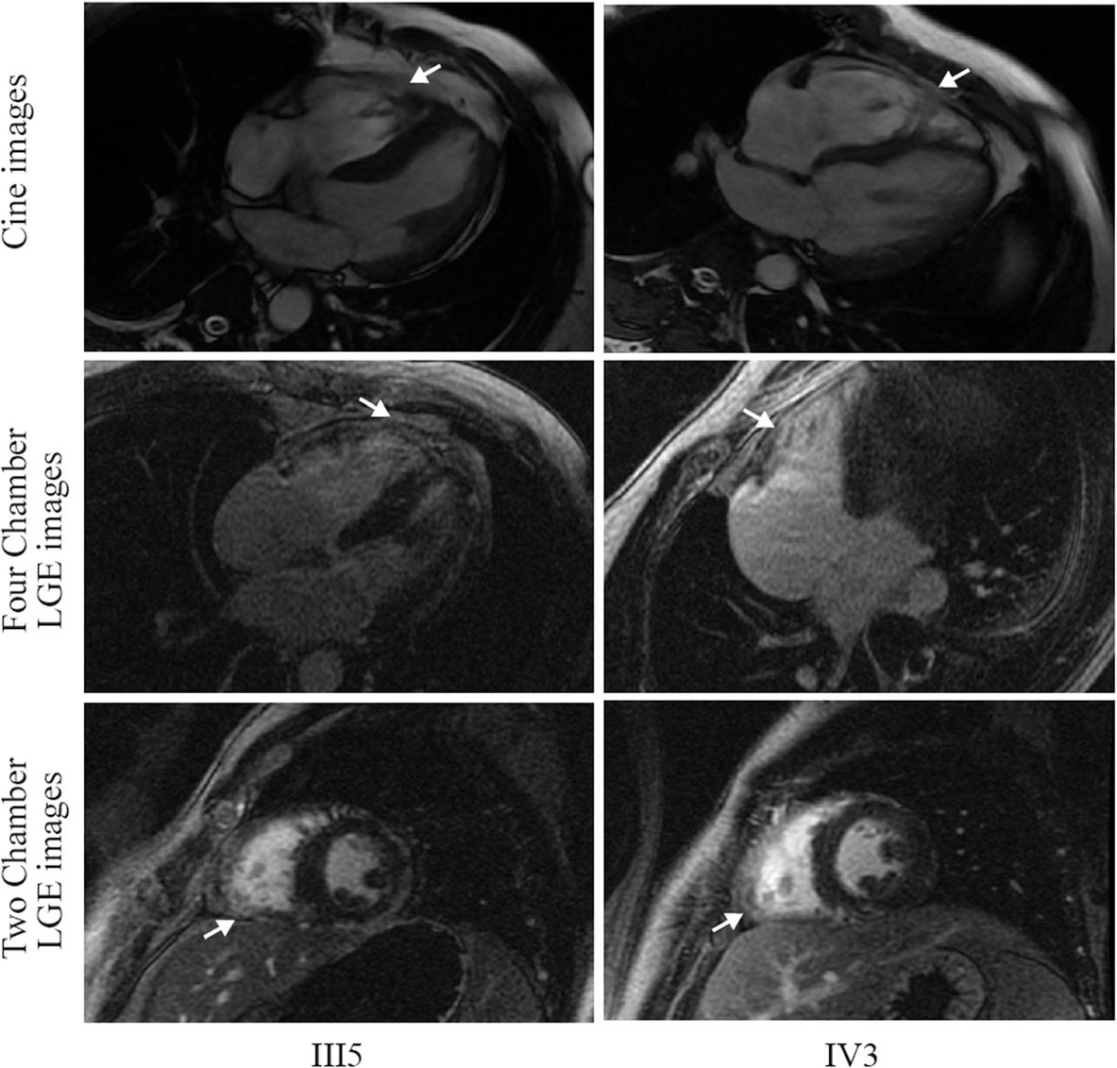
Representative cardiac magnetic resonance images. Myocardium thinning and fatty infiltration (arrow) in the right ventricular and positive bi-ventricular late gadolinium enhancement were detected in III5 and IV3. Myocardium thinning and fatty infiltration (arrow) were detected in the right apical region in III-3. LGE, late gadolinium enhancement
